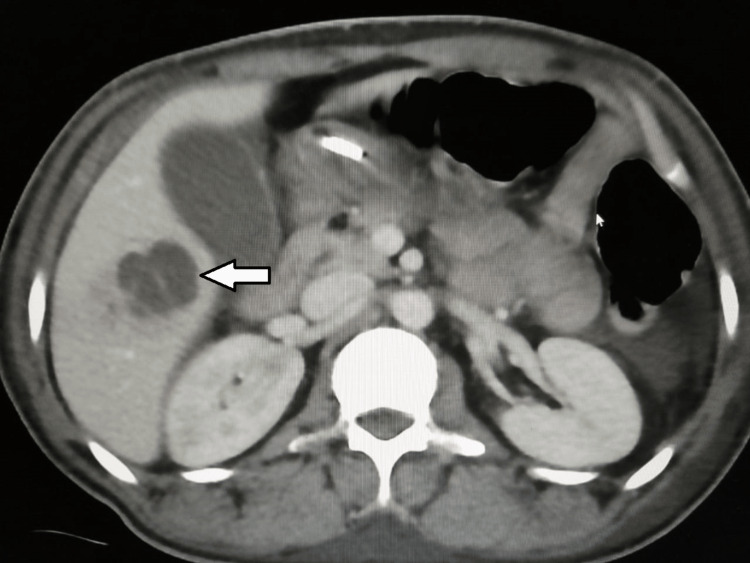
Abdominal CT scan The scan shows a septated cystic lesion in segment 5/6 of the liver (white arrow).
